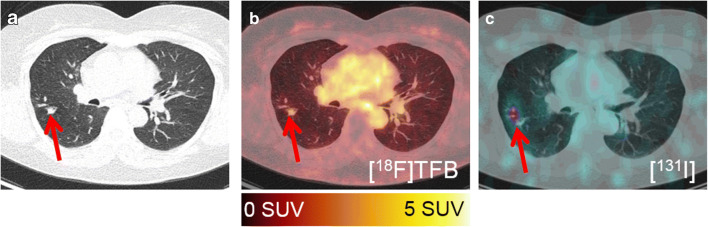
Detection of lung metastasis. A lung metastasis (arrow) of patient #23 (56 years; TG, 5.21 ng/ml; antiTG, < 15 IU/ml; papillary DTC) is detected by CT (a), [^18^F] TFB-PET-CT (b), and [^131^I]iodine SPECT-CT (c)
